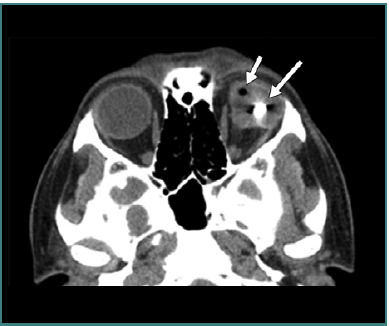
Axial computed tomography scan without contrast of left open globe. The small arrow represents intraocular air and the large arrow represents the metallic intraocular foreign body. Image reproduced with permission from the American Roentgen Ray Society: Yuan WH, Hsu HC, Lin YY, Liao SW. CT of globe rupture: analysis and frequency of findings. AJR Am J Roentgenol. 2014;202(5):4-5. ^©^ 2014 American Roentgen Ray Society.
